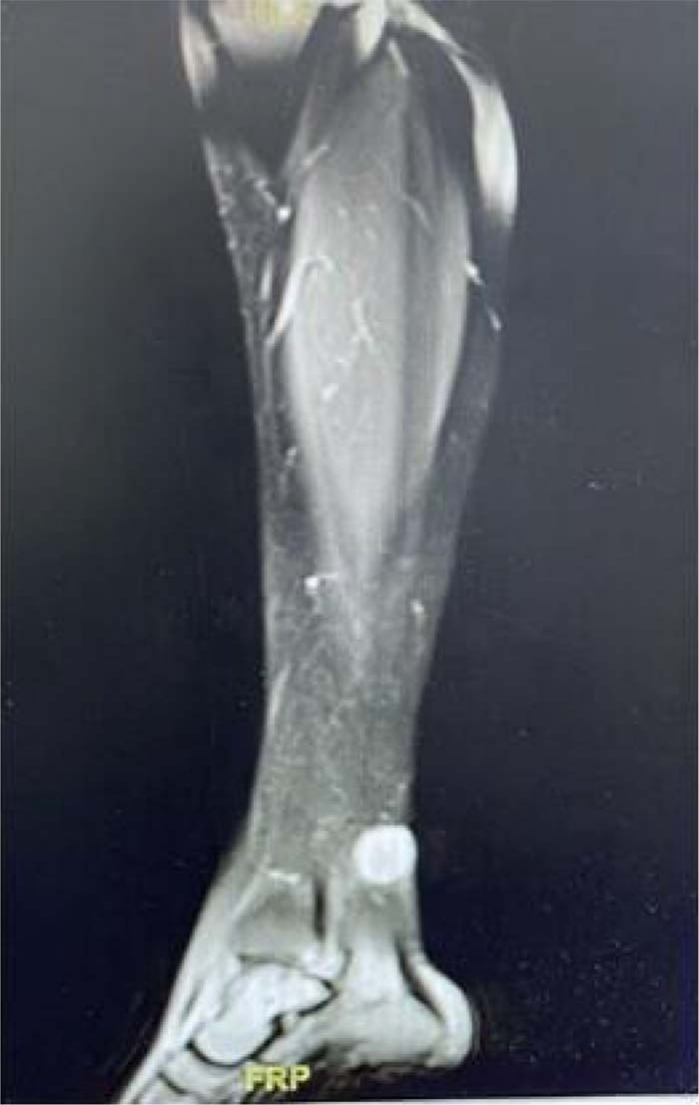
MRI demonstrating subcutaneous soft tissue mass on the posterior aspect of the lower left leg.
